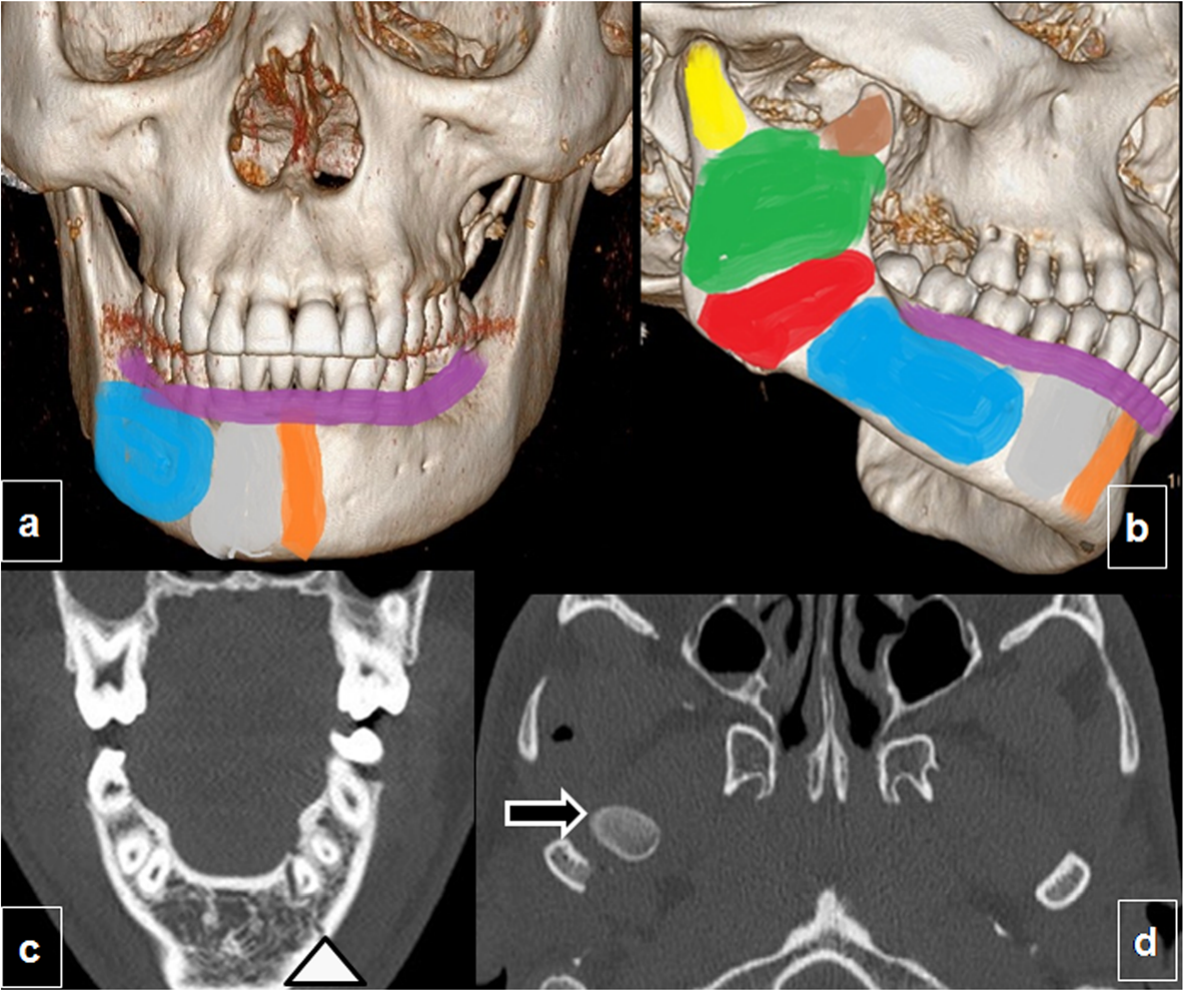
Fractures of the mandible. Anatomy (a, b): three-dimensional CT image with color overlays indicating the parts of the mandible in coronal and lateral oblique orientations: the alveolar process (purple), symphysis (orange), parasymphyseal region (gray), body (blue), angle (red), ramus (green), coronoid process (brown), and condyle (yellow). c, d Bifocal mandibular fracture. c Coronal and (d) axial unenhanced CT of the mandible shows the classical pattern of bifocal mandibular fractures, affecting the body of the mandible on one side (arrowhead in c) and the contralateral condyle with accompanying luxation (arrow in d)
